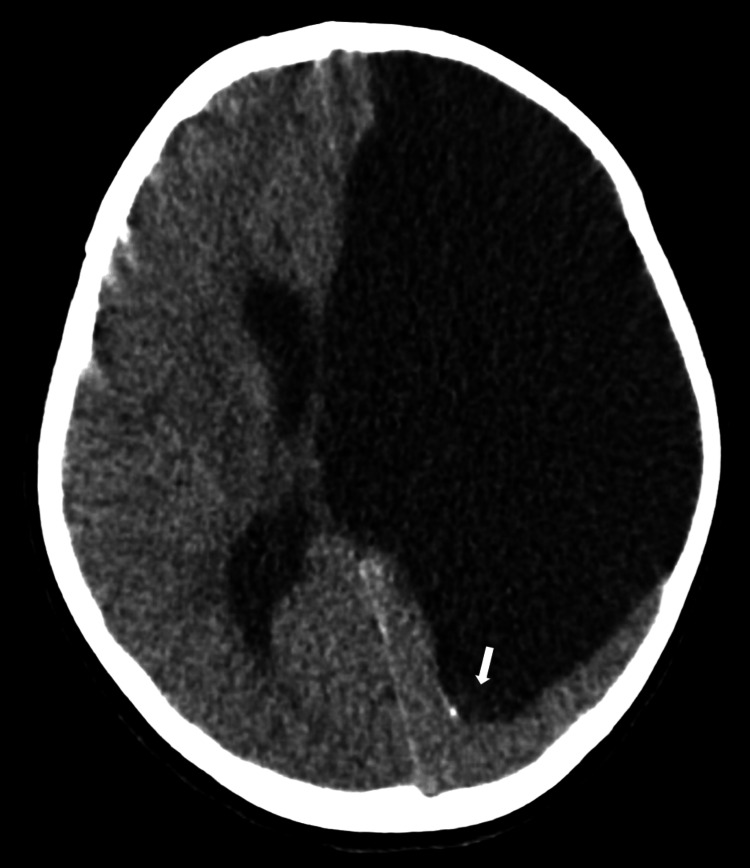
Axial non-contrast CT head nine days after the initial presenting CT head. The image shows near complete resolution of the dependent hematoma (arrow).
